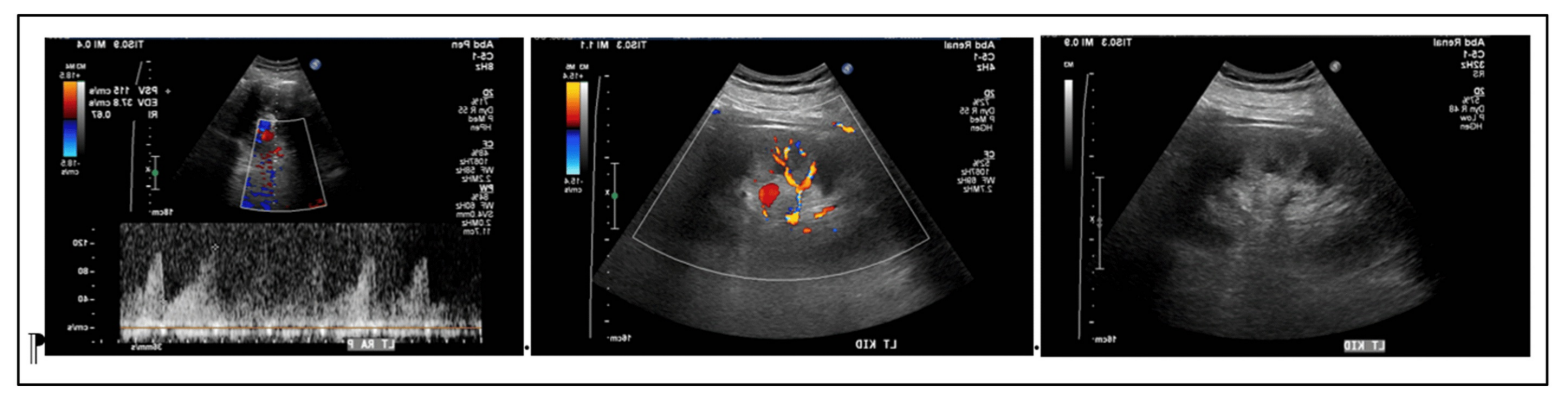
Left kidney ultrasound examination and left renal artery and vein duplex examination on post-operative day one.
